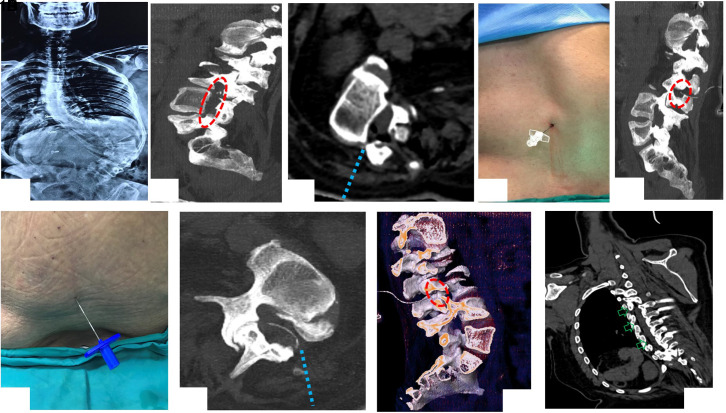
(A) Patient 1—x-ray of lumbar epidural scoliosis, (B) Patient 1—CT scan sagittal section depicting inter-vertebral foraminal defect (red dotted circle-proposed needle Tuohy entry), (C) Patient 1—CT scan axial section demonstrating the proposed needle entry (blue-dotted line) through the intervertebral foramina, (D) Patient 1—Tuohy needle insertion on the left of the spinous process and convex side of the scoliosis, (E) Patient 1—Catheter depicted entering the intervertebral foramina beneath the pedicle (red-dotted circle), (F) Patient 2—Tuohy needle insertion on the right of the spinous process and convex side of the scoliosis, (G) Patient 2—CT scan axial section demonstrating the proposed needle entry (blue-dotted line) through the inter-vertebral foramina and with the catheter entering from inter-vertebral foramina into the epidural space, (H) Patient 2—A volume rendering technique of CT scan depicting catheter entering the intervertebral foramina beneath the pedicle (red-dotted circle), (I) Patient 2—Hypoechoic linear distribution (air) along the convex side of the scoliosis demonstrating the epidural path (green arrows).
